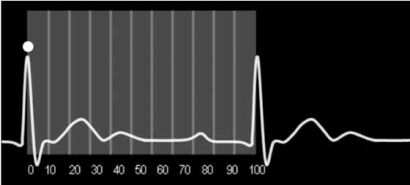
The R-R interval can theoretically be divided into 100 segments for the purposes of cardiac CT.  The first R wave (circle) is the 0% time point at end diastole; the next R wave is the 100% time point of the first cardiac cycle and the 0% time point of the next cardiac cycle.
